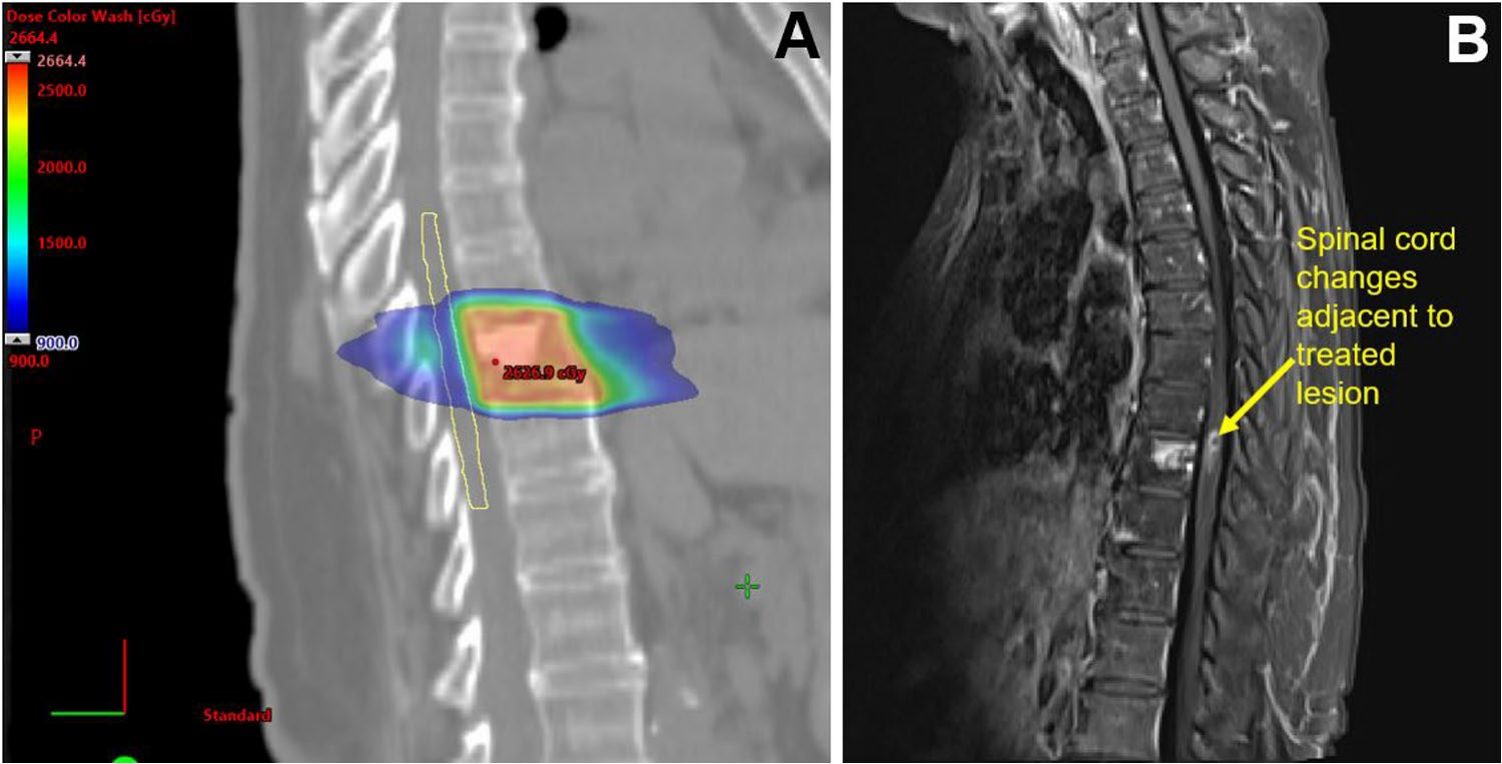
A A sagittal image of the dose distribution of a patient that received stereotactic body radiation therapy (SBRT) to a dose of 24 Gy in 2 fractions to the T9 vertebral body. The spinal cord contour is shown in yellow. B A post-treatment MRI demonstrating abnormal enhancement in the spinal cord adjacent to the treated vertebral body that was determined on serial imaging to represent SBRT-related changes as opposed to recurrent disease
